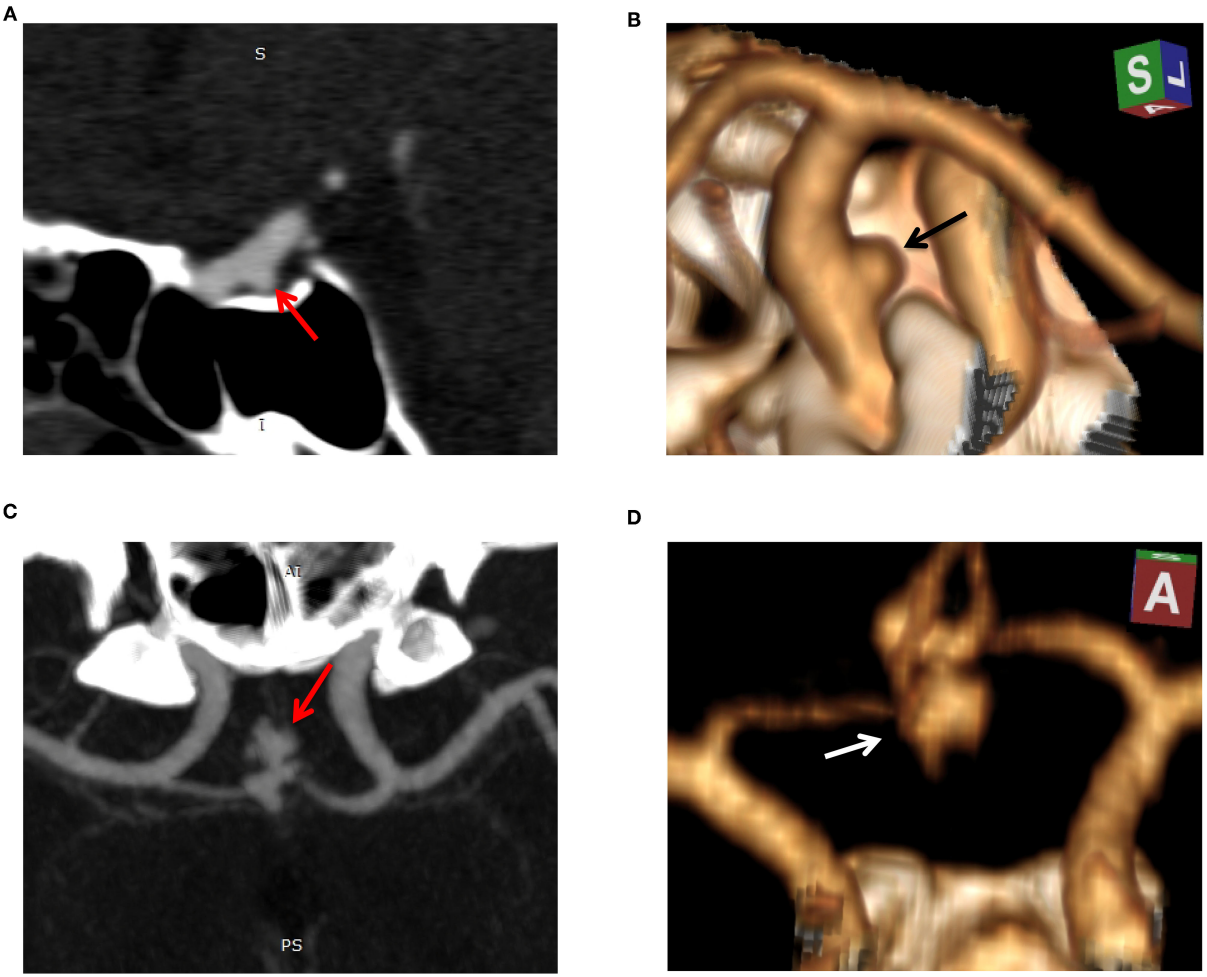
Example cases of unruptured and ruptured aneurysm features. Case 1: sagittal reconstructed maximum intensity projection (MIP, A) and 3D volume-rendered (3D-VR) CT angiography (CTA, B) images of a 52-year-old-male with unruptured aneurysm. The aneurysm was small (3.5 mm in maximal diameter), regular, and located on the internal carotid artery. Radiomics score was −6.1 indicating a low risk of rupture. Case 2: MIP (C) and 3D-VR CTA (D) images of a 50-year-old-male with ruptured aneurysm. The aneurysm measured 9.4 mm in maximal diameter, was irregular (lobulated with multiple daughter sacs), and located on the anterior communicating artery. Radiomics score was 3.5 indicating a high risk of rupture.
